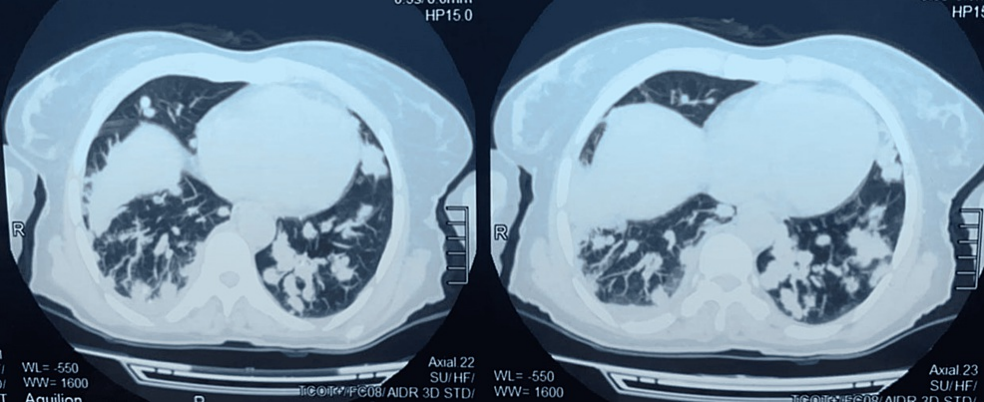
Thoracic CT scan, parenchymal window: multiple rounded lesions of different sizes disseminated to the two pulmonary hemifields
